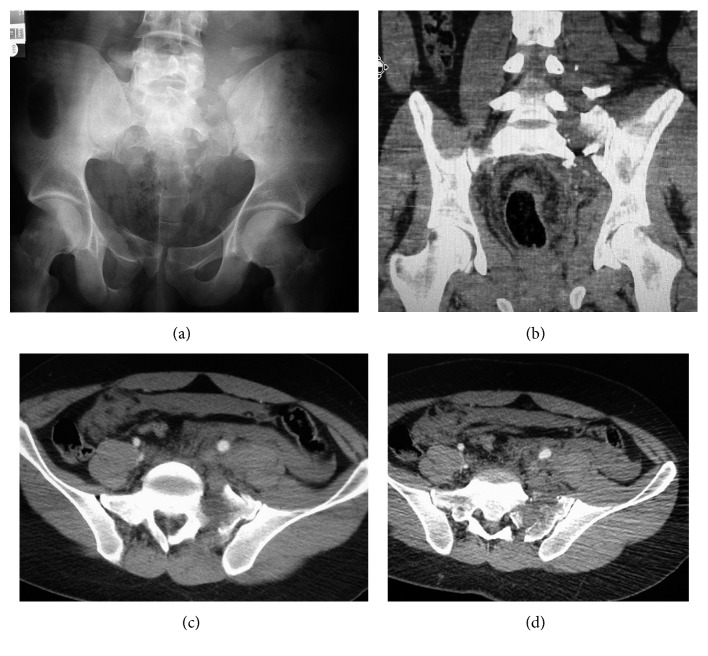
(a) Anteroposterior (AP) radiograph and (b) coronal CT cut demonstrating a comminuted Denis zone 2 sacral fracture with involvement of the sacral ala and fracture of the L4 and L5 transverse processes. (c, d) Axial CT cuts demonstrating the severe comminution involving the left sacral neuroforaminae.
